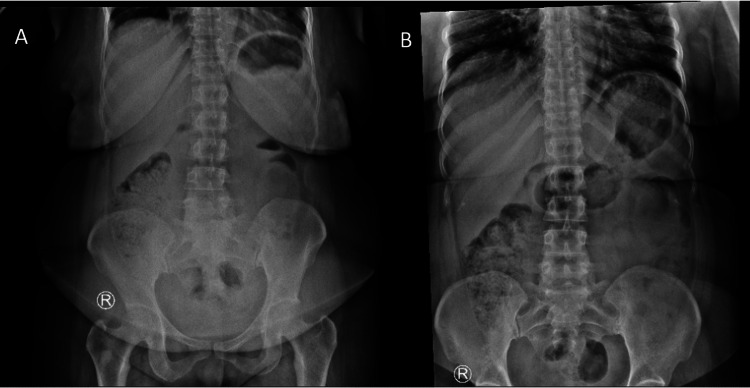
First presentation abdominal X-ray (A) Erect; (B) Supine
